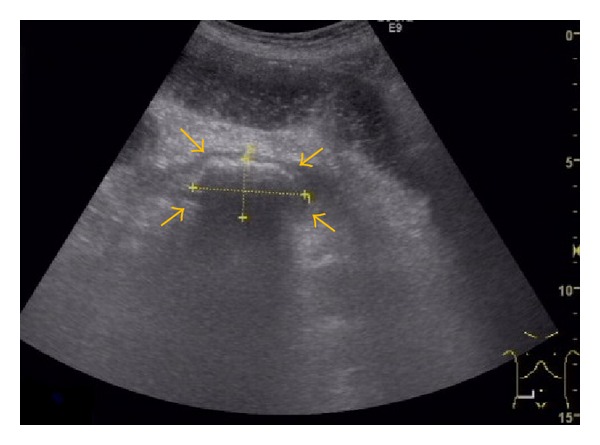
Abdominal ultrasound image on hospital day 59 showing a hyperechoic solid space-occupying lesion (45 × 23 mm) with acoustic shadowing (arrows) in the ileocecal region.
